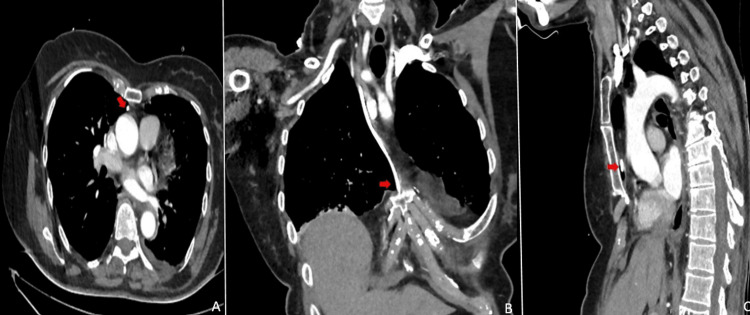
Patient's Contrast-enhanced computed tomography (CECT) Malpositioned port catheter, indicated by red arrows, displayed on multiple planes in chest CECT. A: axial, B: coronal, C: sagittal
